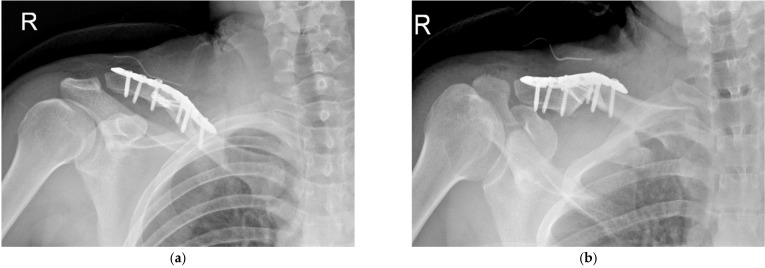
Postoperative right clavicle AP (a) and lordotic (b) X-rays.
